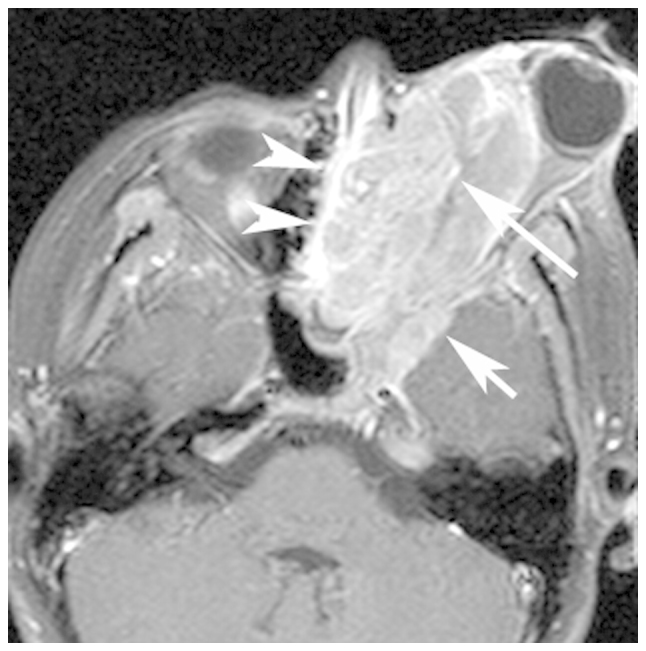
Case 8, a 13-year-old female with embryonal rhabdomyosarcoma in the orbit. Axial contrast-enhanced T1-weighted imaging shows a left orbital mass with homogeneous enhancement (long arrow) extending to the left ethmoid sinus (arrowhead) and left middle cranial fossa (short arrow). The ill-defined tumor had displaced the globe laterally and superiorly.
